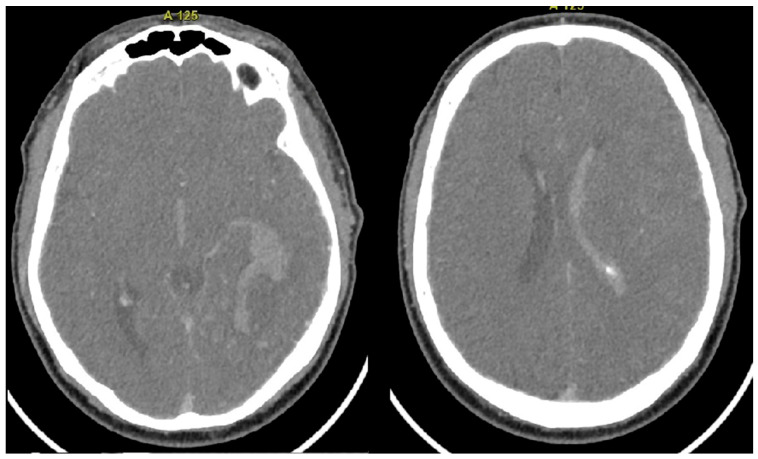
Computed Tomography (CT) Images, Axial View: The images reveal acute intracerebral and intraventricular hemorrhage.
